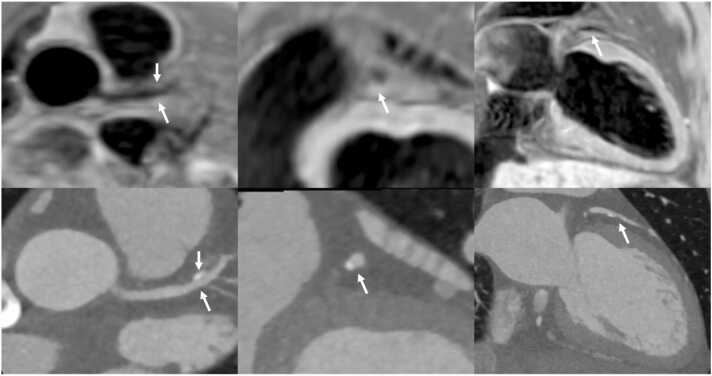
Comparison of iT2prep-BOOST black-blood imaging and CCTA in a patient with partially calcified plaque in the LAD artery. The top row demonstrates black-blood BOOST images in different views, highlighting the hyperintense signal from the partially calcified plaque in the LAD (white arrows). The bottom row shows the corresponding CCTA views of the same vessel. *LAD* left anterior descending, *BOOST* bright‐blood and black‐blOOd phase SensiTive, *CCTA* coronary computed tomography angiography
